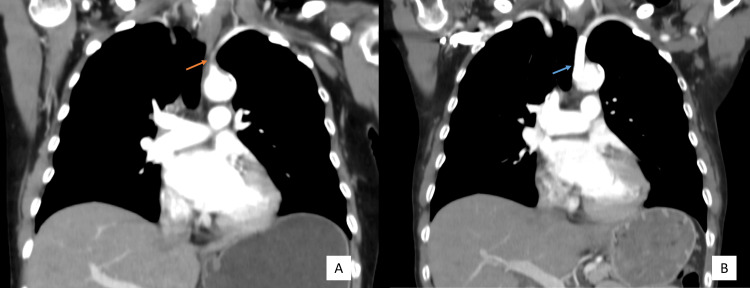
Computed tomography with angiogram showing subclavian stenosis before and after vascular intervention (A) Before, orange arrow indicating left subclavian stenosis. (B) After, blue arrow indicating vascular conduit in the left subclavian artery origin.
